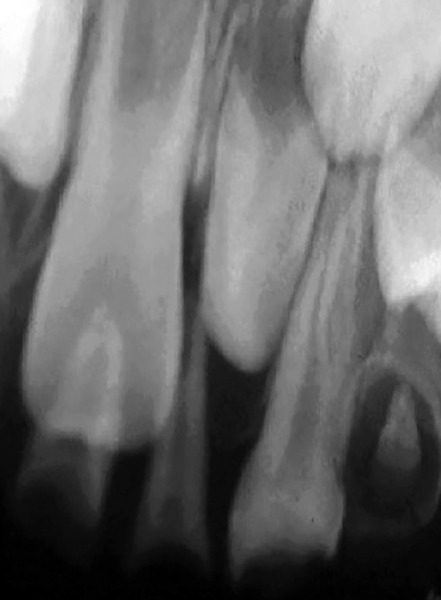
Periapical radiograph showing dens invaginatus in a primary maxillary left first molar
